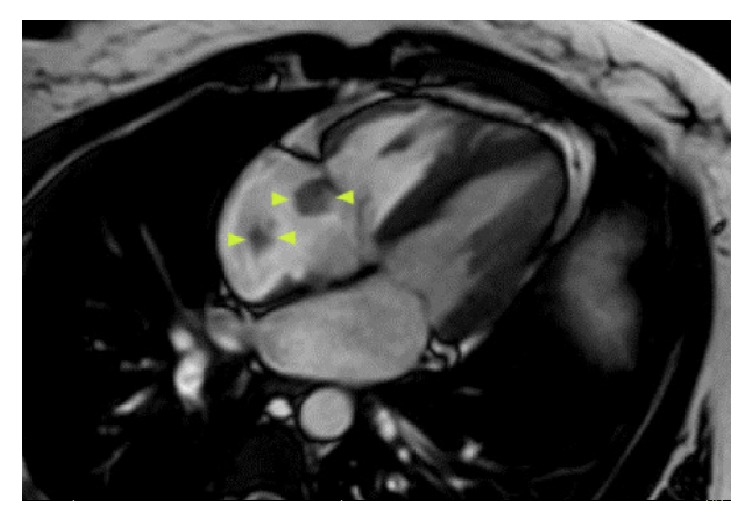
Cardiac MRI image showing multiple right atrial thrombi.
